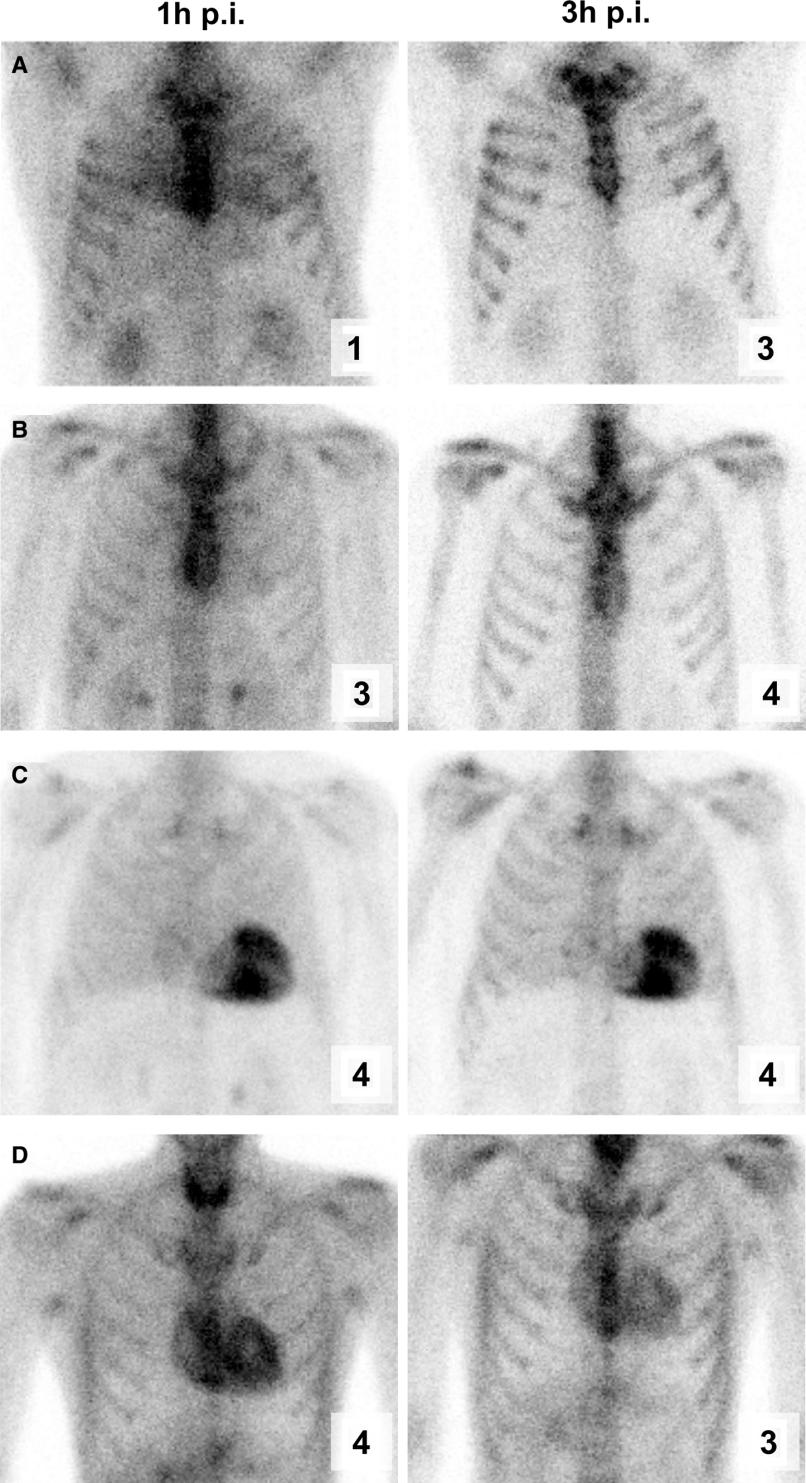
Confidence during visual assessment. Patient examples showing the confidence score (right lower corner) that rated the reader’s confidence in assigning a specific visual score 0-3 (note: not the confidence for the binary decision of a positive vs negative case). Each image is scaled to its individual count maximum. A 53-year-old male with systemic ATTR amyloidosis but cardiac involvement excluded by biopsy. B 42-year-old male with systemic ATTR amyloidosis but echocardiography and clinical status not suggestive of cardiac involvement. C 77-year-old male with biopsy-proven cardiac ATTR amyloidosis. D 39-year-old male with proven systemic hereditary ATTR amyloidosis but repeated echocardiography over 3 years not suggestive of cardiac involvement
